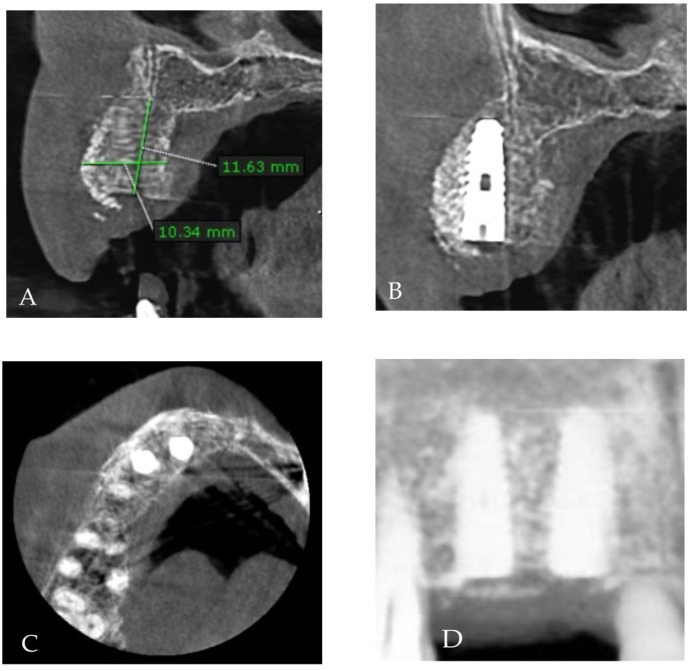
Cone-beam CT images of the implants position in the augmented bone (A), (B) sagittal view, (C) axial view, and (D) coronal view.
